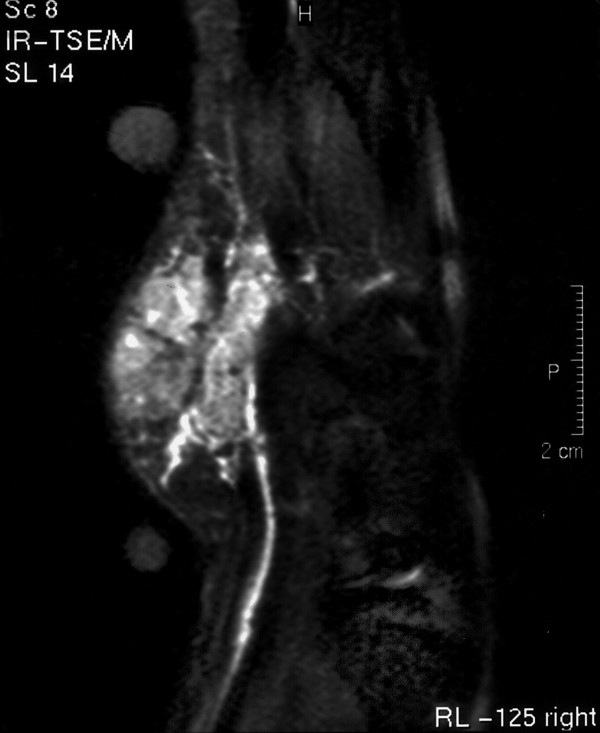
MRI of the right hand showing a pseudosarcomatous mass encircling the ulnar artery.
